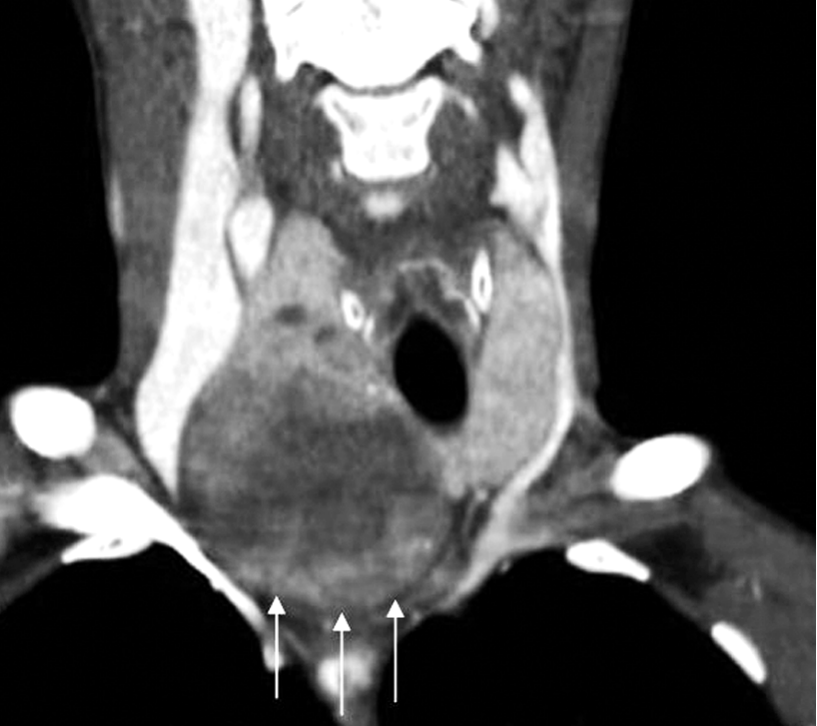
Contrast enhanced CT scan with coronal reconstructed image: right lobe of the thyroid gland shows an inhomogeneous mass with focal areas of hemorrhage. Compression and deviation of the trachea is also present.
